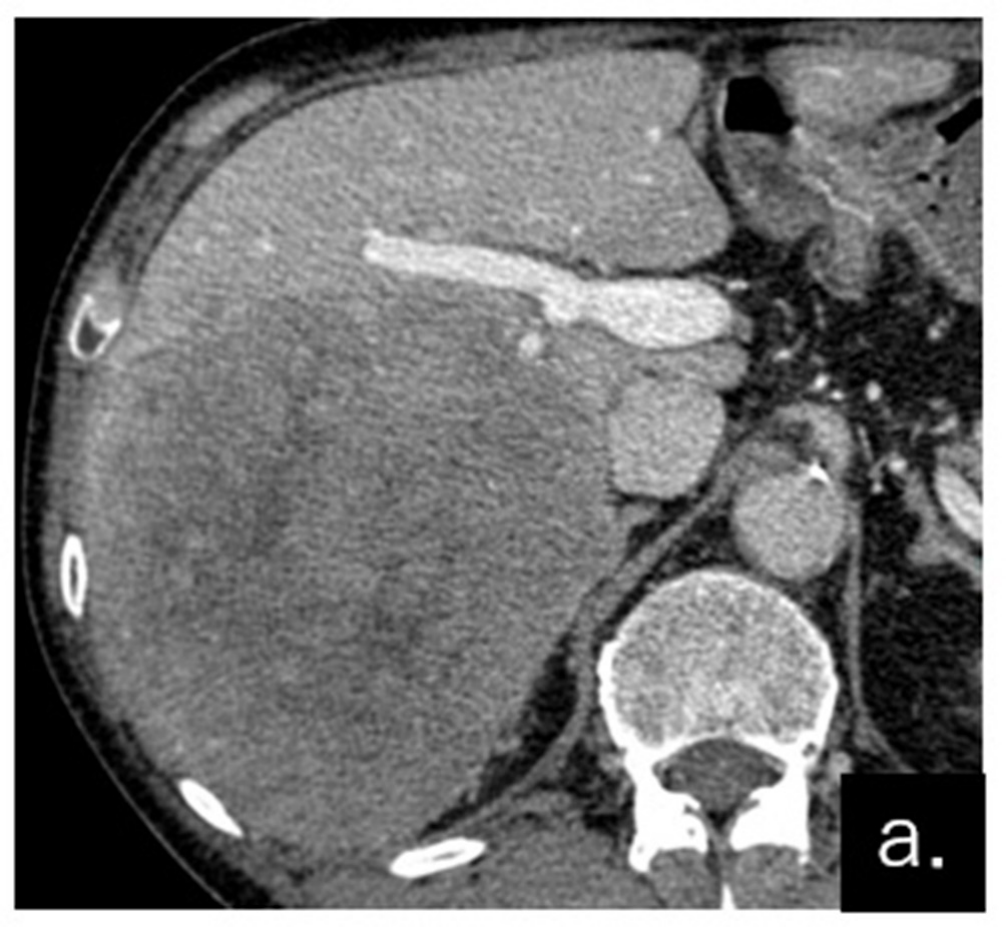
Contrast-enhanced CT (CECT) of the liver before hepatectomy. The primary tumor (hepatocellular carcinoma) occupied the right lobe of the liver.
